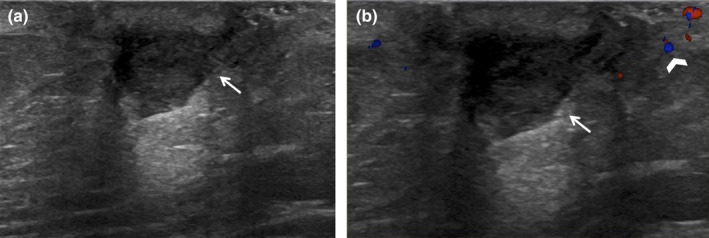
49‐year‐old man with a previous right nipple piercing presented due to a painful palpable lump within the right subareolar region. Ultrasound evaluation was performed with a ML6‐15 MHz transducer at a frequency of 15 mHZ and the colour gain set to 5 cm/s. Greyscale (a) and colour (b) images showed a hypoechoic cystic collection (arrow) directly behind the nipple with minimal surrounding echogenicity and vascularity (chevron).
